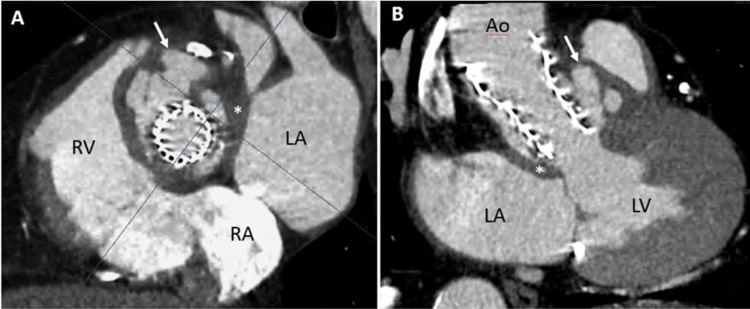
Cardiac CT scan Aortic periprosthetic false aneurysm (white arrow) suggestive of a circulating abscess. (A) Aortic cross-sectional plane showing a multifocal circulating periprosthetic image around the aortic root. (B) Reformatted three-chamber view showing the expansion of the circulating mass during systole CT: computed tomography; RV: right ventricle; RA: right atrium; LA: left atrium; Ao: ascending aorta
